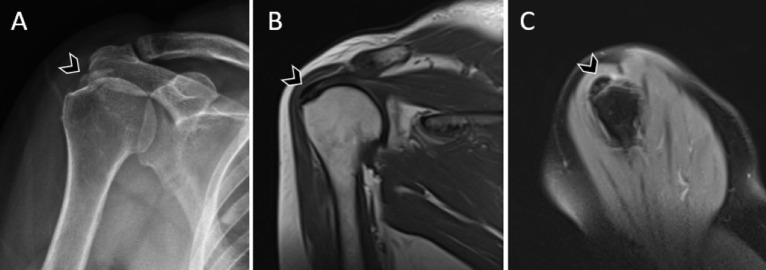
Calcific tendinitis of the supraspinatus tendon without an associated tear. Right shoulder images of a 50-year-old female patient. (A) Plain radiograph of the right shoulder demonstrates an approximately 15 mm calcific radiopaque focus at the supraspinatus tendon insertion (arrowhead). (B) Coronal T1-weighted magnetic resonance imaging shows a corresponding low-signal-intensity calcific focus at the same location (arrowhead). (C) Sagittal proton density–weighted image clearly demonstrates the calcific deposit (arrowhead), with no definite evidence of a supraspinatus tendon tear (arrowhead).
